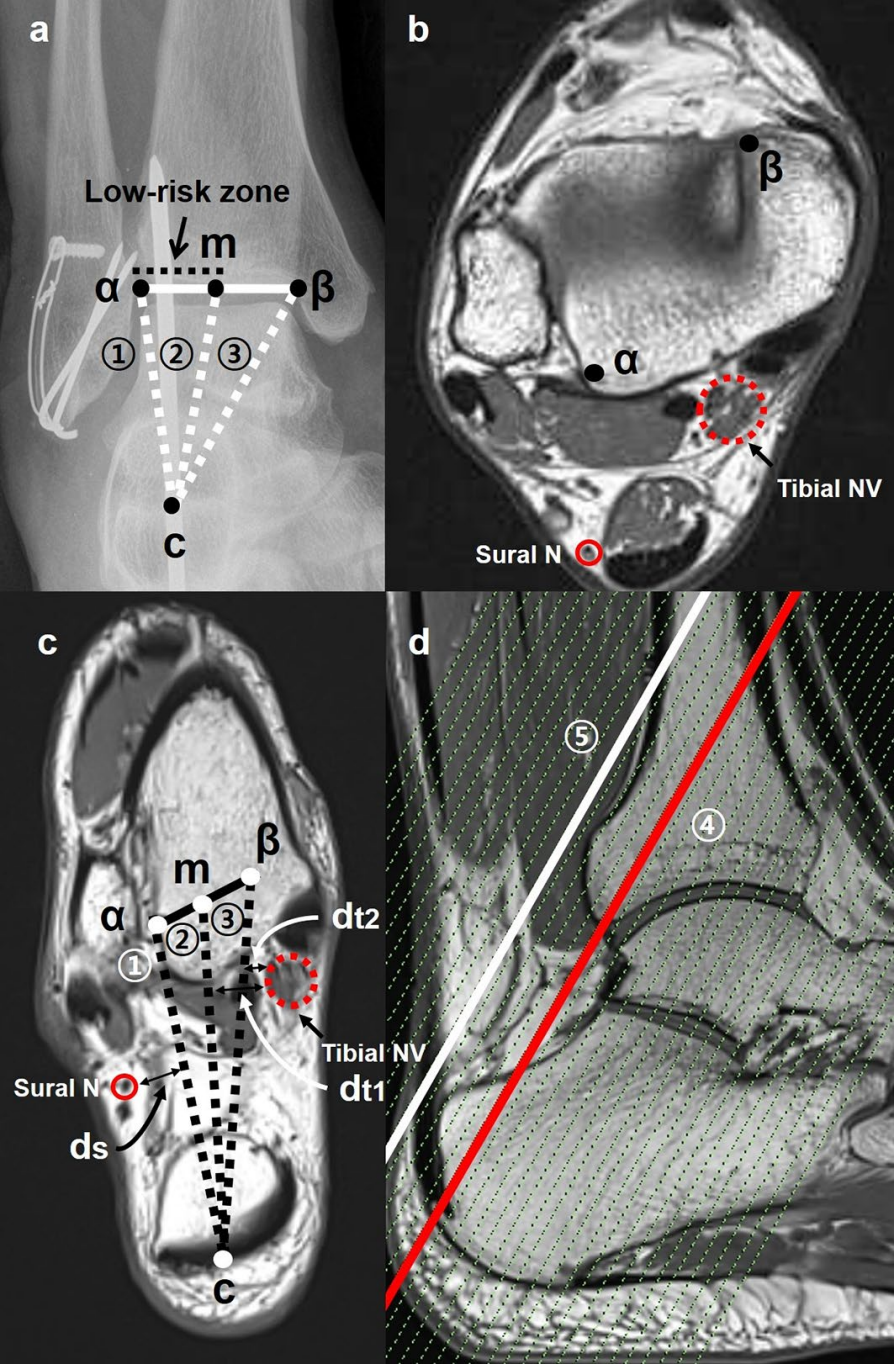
(a) The C-arm fluoroscopic ankle mortise radiograph is used during pin insertion. The low-risk zone on the simple radiograph was transferred to the MRI to measure the distance between its borders and the neurovascular structures. (b) On the axial image, the lateral end of the posterior malleolus at the joint level (α) and the anteromedial end of the anterior distal tibial articular surface (β) are marked. The marks can be shown in every oblique axial slice using Pictured Archives and Communication System (PACS). (c)The oblique axial slice, oriented on the trajectory of the pin fixation (from the center of the calcaneal tuberosity to the distal tip of the posterior malleolus: ④), was selected for the measurement. A line was drawn connecting the two ends (α, β). Then, a line was drawn from the center of the calcaneal tuberosity (c) bisecting (m) the line connecting the two ends (α, β), indicating the medial border of the low-risk zone (②). Also, for the low-risk zone’s lateral border (①), a line was drawn from the center of the calcaneal tuberosity (c) to the lateral end of the posterior malleolus (α). Similarly, for the most medial border (③), a line was drawn from the center of the calcaneal tuberosity (c) to the anteromedial end of the anterior distal tibial articular surface (β), allowing us to confirm the safety of aiming the pin toward the medial end of the anterior distal tibial articular surface during fixation. The three borders of the low-risk zone are observable in every oblique slice using PACS. The distance (d_s_) between the sural nerve (closed circle) and the lateral border of the low-risk zone (①), (d_t1_) the posterior tibial neurovascular structures (dotted circle) and the medial border of the low-risk zone (②), and (d_t2_) the posterior tibial neurovascular structures and the most medial border of the low-risk zone (③) were measured. (d) Five additional consecutive oblique axial slices within 1 cm proximal from the distal tip of the posterior malleolus (④) were recorded to determine the safety of inserting the pin into the posterior malleolus 1 cm proximal from the distal tip (⑤).
